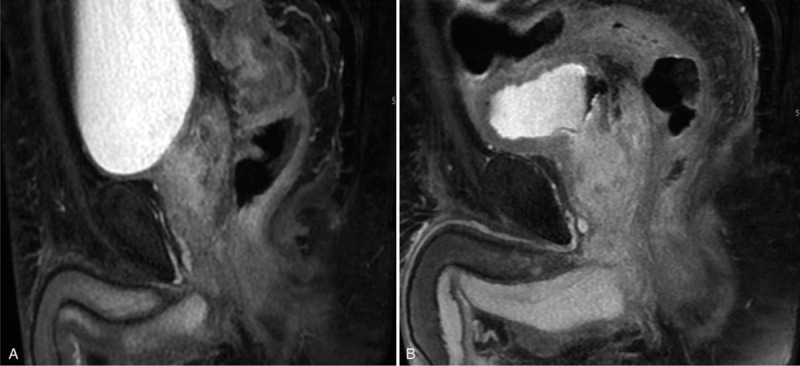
(A and B) MRI findings after neoadjuvant chemoradiation therapy.
